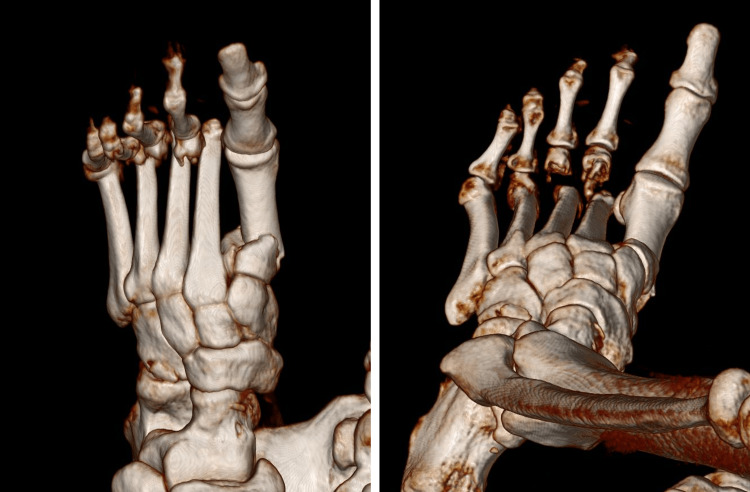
Three-dimensional reconstruction of the left foot's computed tomography Three-dimensional reconstruction of the left foot's computed tomography showing left foot first metatarsal proximal fracture, left foot second, third, fourth, and fifth metatarsal distal displaced fractures, left foot cuboid, and navicular bone fractures. The patient's left foot's metatarsals were all fractured.
